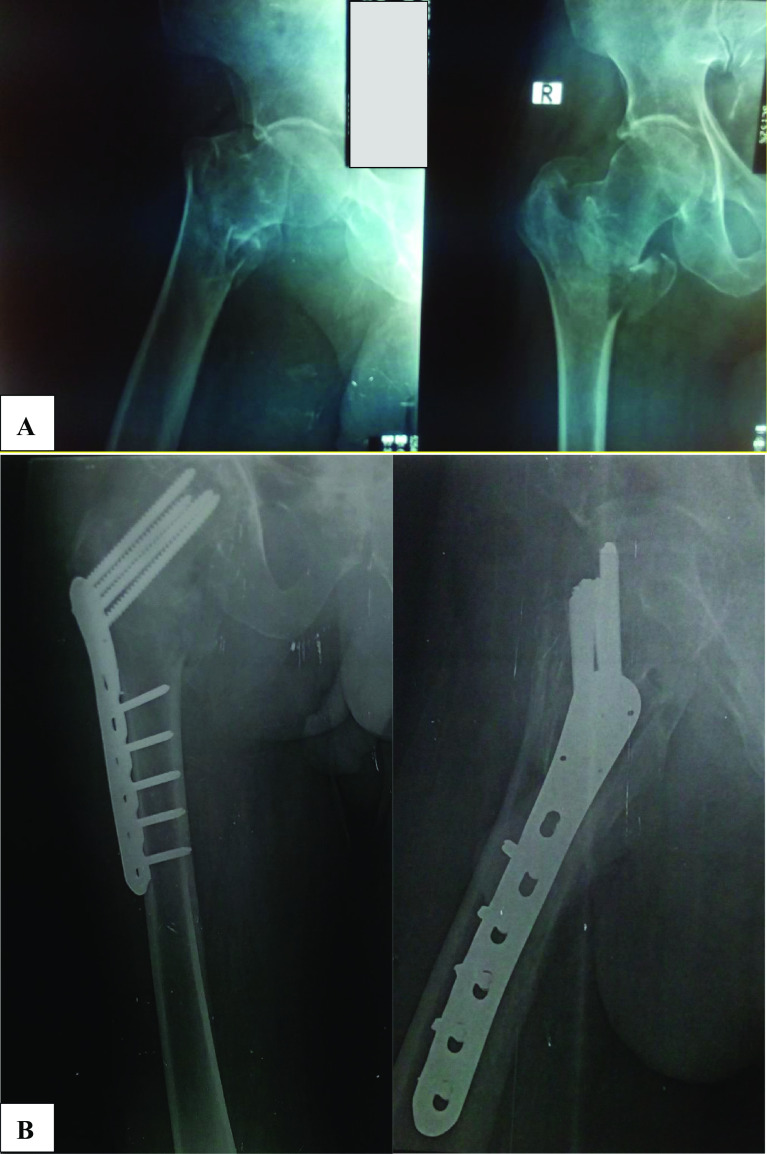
X-rays of 62-year-old male patient with AO 31A2.2 pertrochanteric fracture managed by PFLP; (A) Pre-operative X-rays; (B) X-rays at 6 months follow-up.
